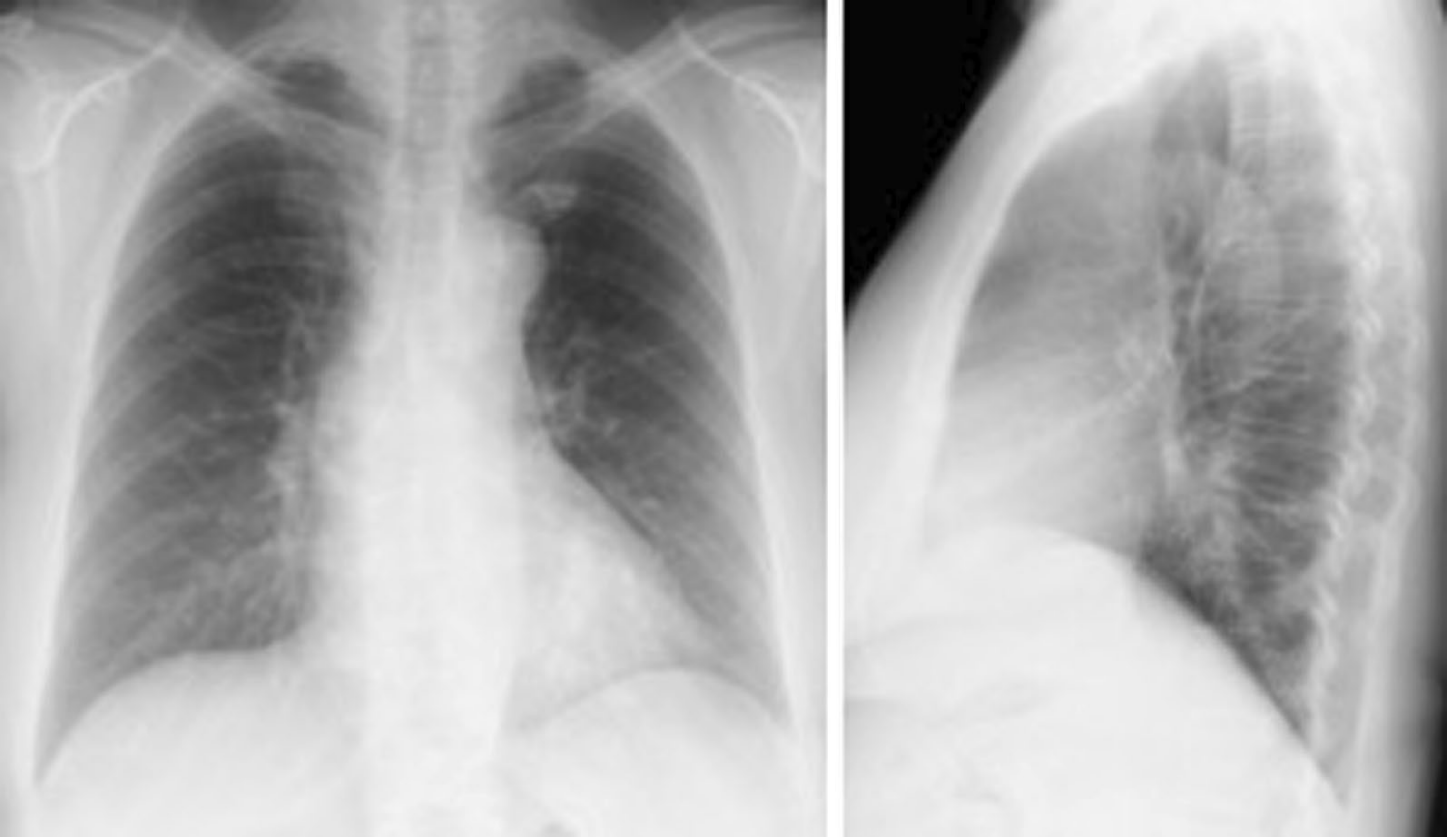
Chest x-ray taken when the patient was admitted to the hospital
